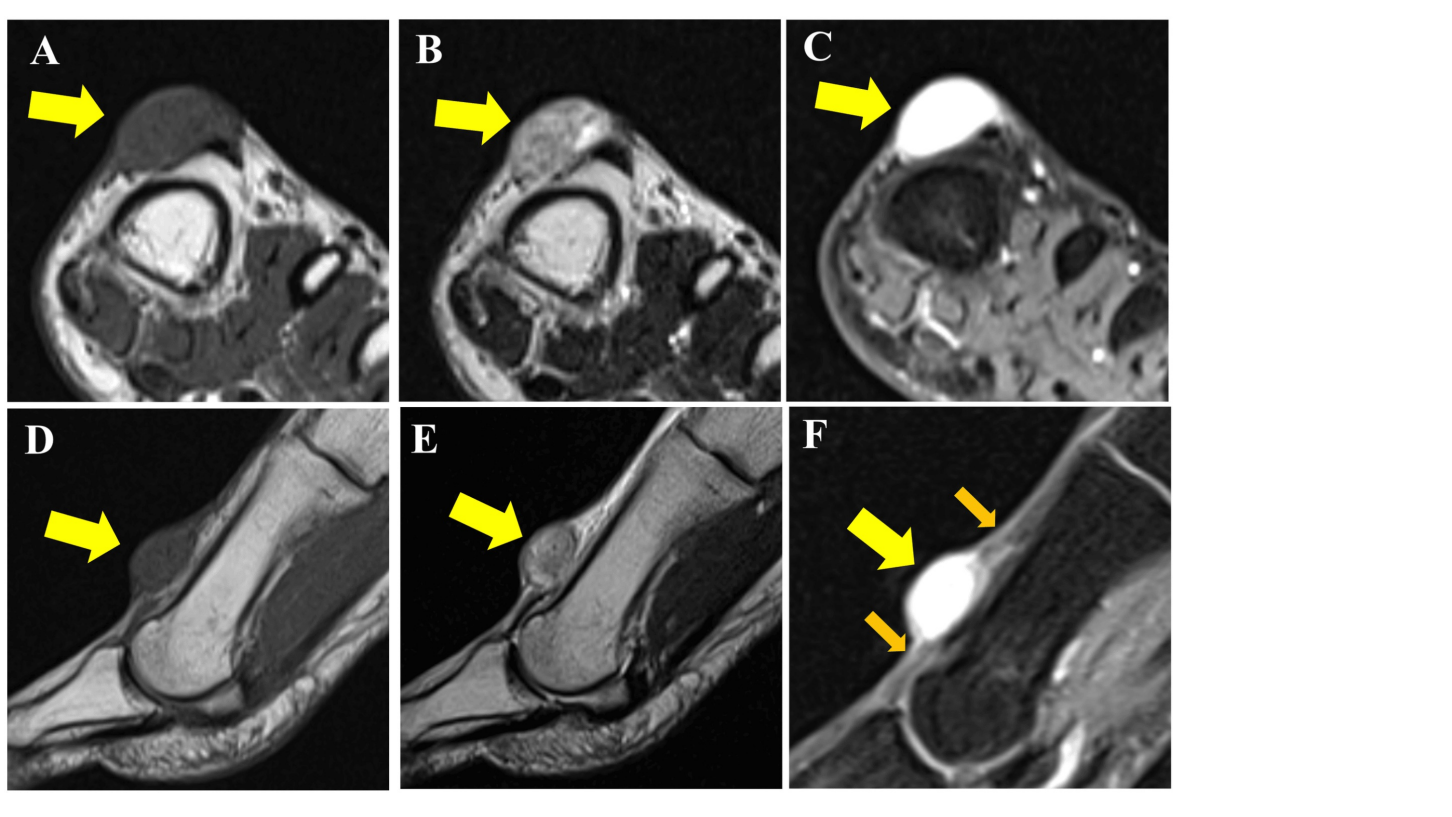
MRI findings Axial (A-C) and sagittal (D-F) views. There is a well-defined subcutaneous mass with a clear boundary and no invasion into bone. Low signal intensity on T1-weighted imaging (A, D, yellow arrow), heterogeneous high signal intensity on T2-weighted imaging (B, E, yellow arrow), and avid enhancement on contrast-enhanced T1-weighted imaging (C, F, yellow arrow). The proximity between a blood vessel and the tumor (F, orange arrows)
